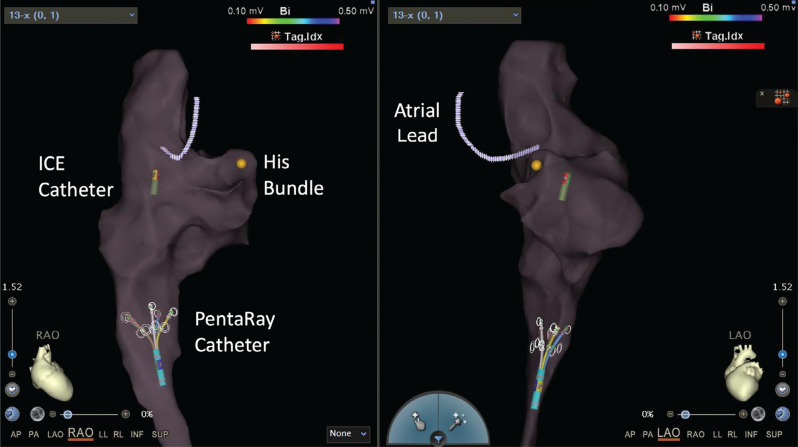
Incorporation of the course of the right atrial lead as shown on intracardiac echocardiography (Figure 11) into the electroanatomic map. *Abbreviation:* ICE, intracardiac echocardiography.
